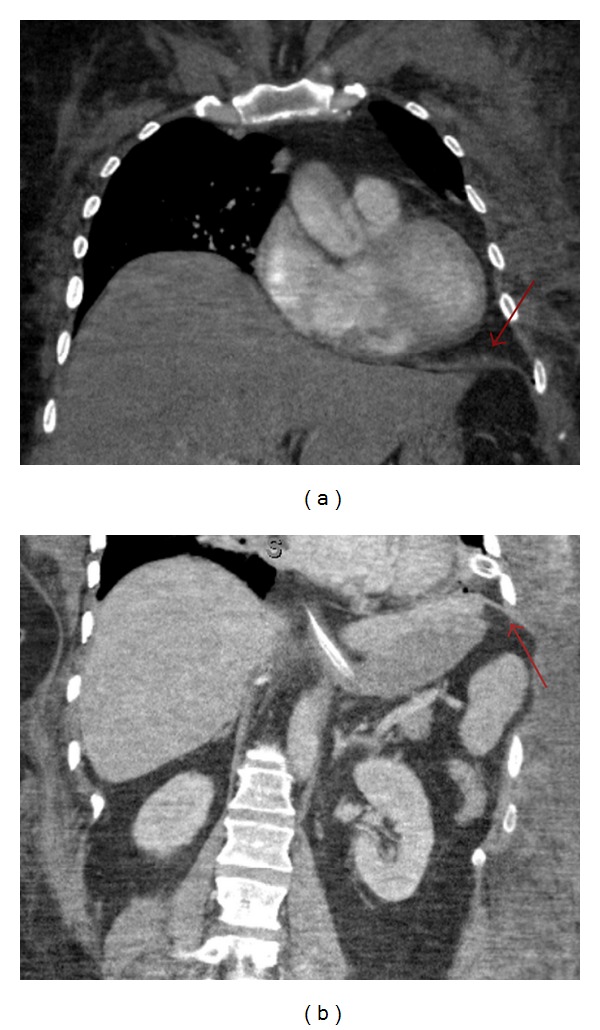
Coronal CT images of the chest and abdomen taken 1 week after surgical repair of the diaphragm (red arrow) show no residual transdiaphragmatic or intercostal herniation.
